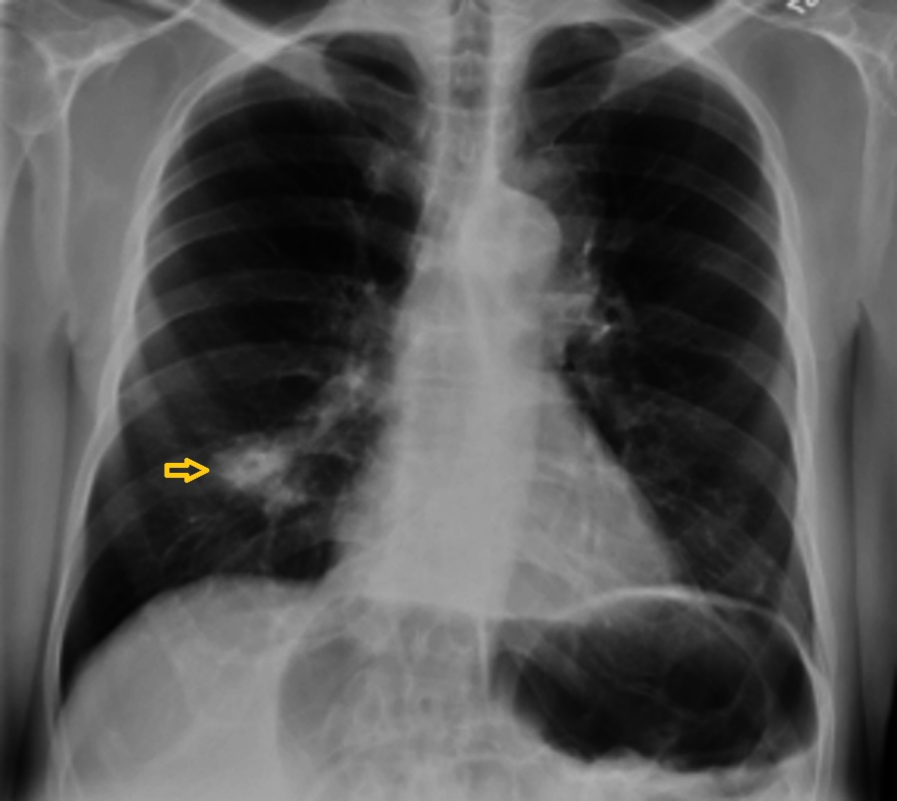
Chest X-ray showing right middle lobe opacity (arrow).
